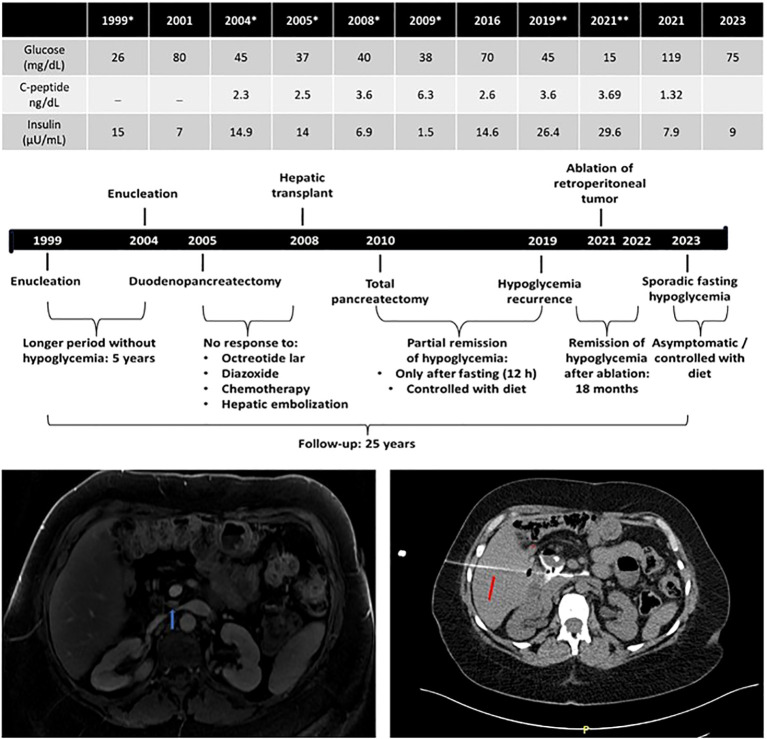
Long-term outcome (25 years) of a patient (case 4) diagnosed with malignant insulinomatosis 7 years after multiple surgical treatments by recurrent/persistent hypoglycemia. *, basal biochemical/hormonal values documenting hyperinsulinemic hypoglycemia before surgical procedures: 1999, enucleation; 2004, enucleation; 2005; subtotal duodenopancreatectomy; 2008, liver transplant after biopsy confirming hepatic metastasis and unsuccessful multiple treatments (diazoxide, somatostatin analogs, chemotherapy, and hepatic embolization); 2019/2021, basal biochemical/hormonal values documenting hyperinsulinemic hypoglycemia before radiofrequency ablation (red arrow: needle of ablation) of retroperitoneal tumoral mass (blue arrow) detected by magnetic resonance imaging in 2021.
